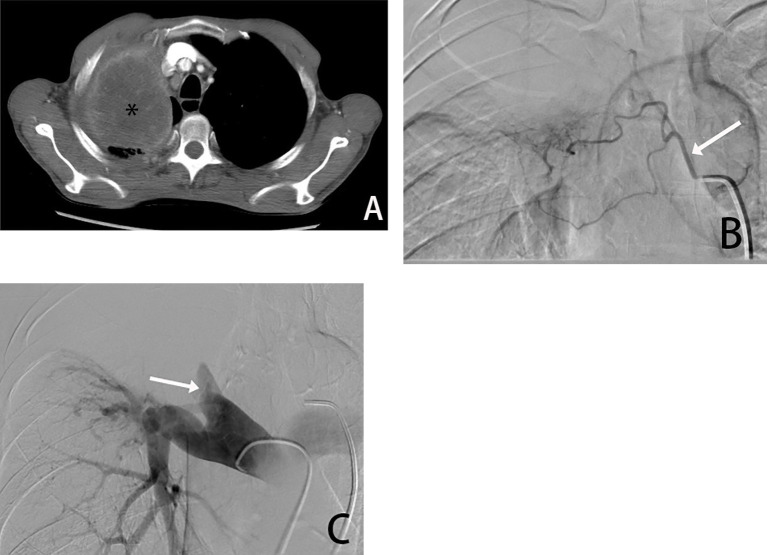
Male, 66 years old, tumor found in the right upper lung due to chest pain, biopsy pathology diagnosed squamous cell carcinoma. (A) is a CT transverse image showing a right upper lung tumor(*). (B) shows a right bronchial arteriogram (→) showing that the right lung tumor is supplied by the right bronchial artery, and (C) is a pulmonary arteriogram showing compressive violation of the right upper pulmonary artery, which is not involved in the blood supply.
